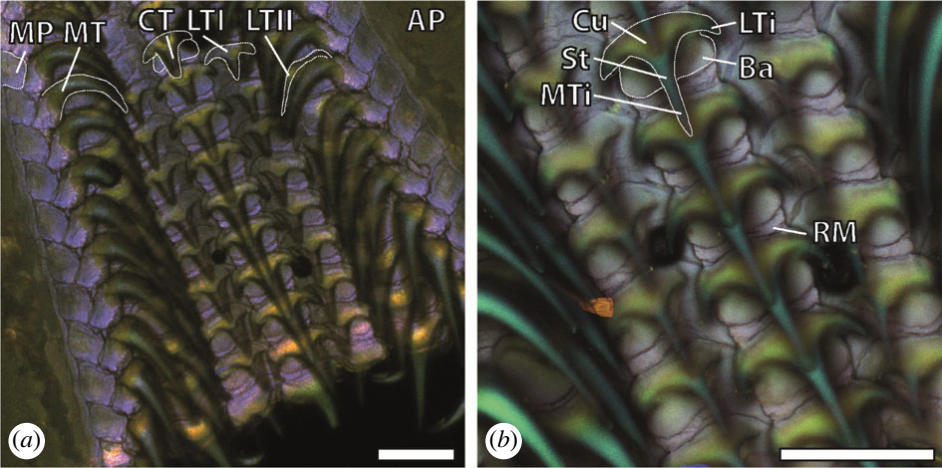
CLSM images of one radula showing the heterogeneities of the material. (*a*) Overview of region 60–90%. (*b*) Magnification of region 80–90%. Scale bars, 180 μm. AP, alary processus (= hyaline shields); Ba, basis; CT, central tooth; Cu, cusp; LTI, lateral tooth I; LTII, lateral tooth II; LTi, lateral tip; MP, marginal plate; MT, marginal tooth; MTi, major tip; RM, radular membrane; St, stylus.
